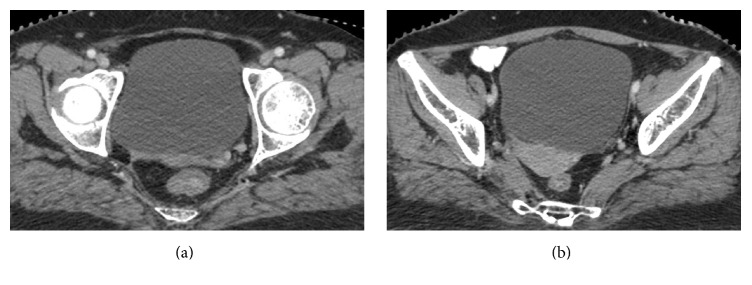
CT scan after six cycles of chemotherapy showing almost complete regression of the cervical mass and its extensions (a, b).
